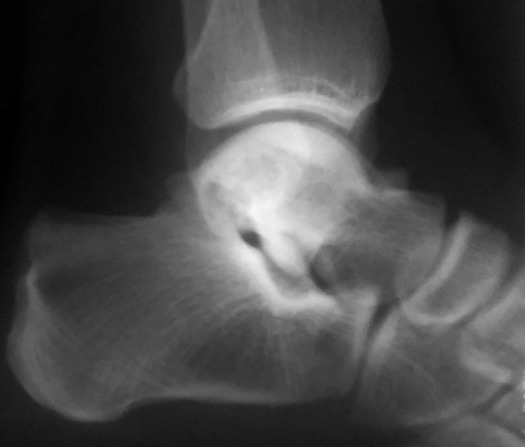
Aspect radiographique d'une proéminence de l'angle postéro-supérieur du calcanéum
